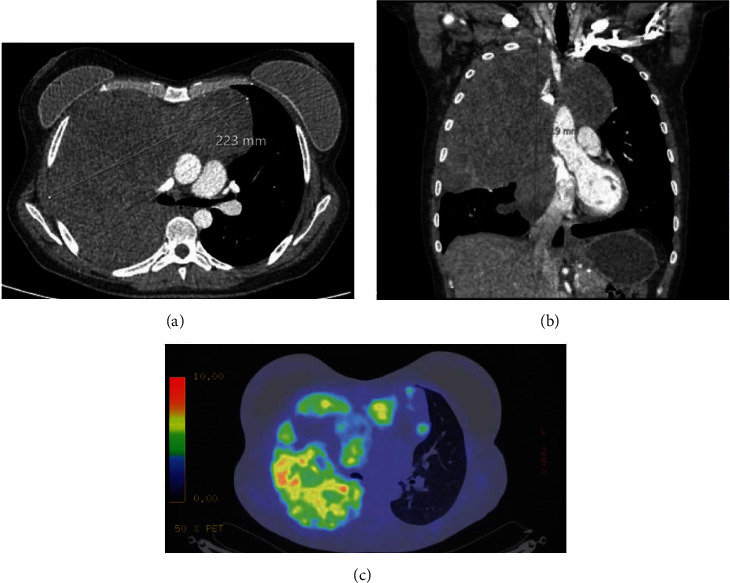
(a) Axial and (b) coronal computed tomography of the thorax showing a tumour invading the right lung, occupying most of the right hemithorax, and crossing the midline to the left side of the mediastinum. (c) ^18^F-fluorodeoxyglucose positron emission tomography integrated with computed tomography showing heterogeneous radiotracer uptake in the tumour, with maximum standardized uptake value of 13.1 and areas of photopenia.
